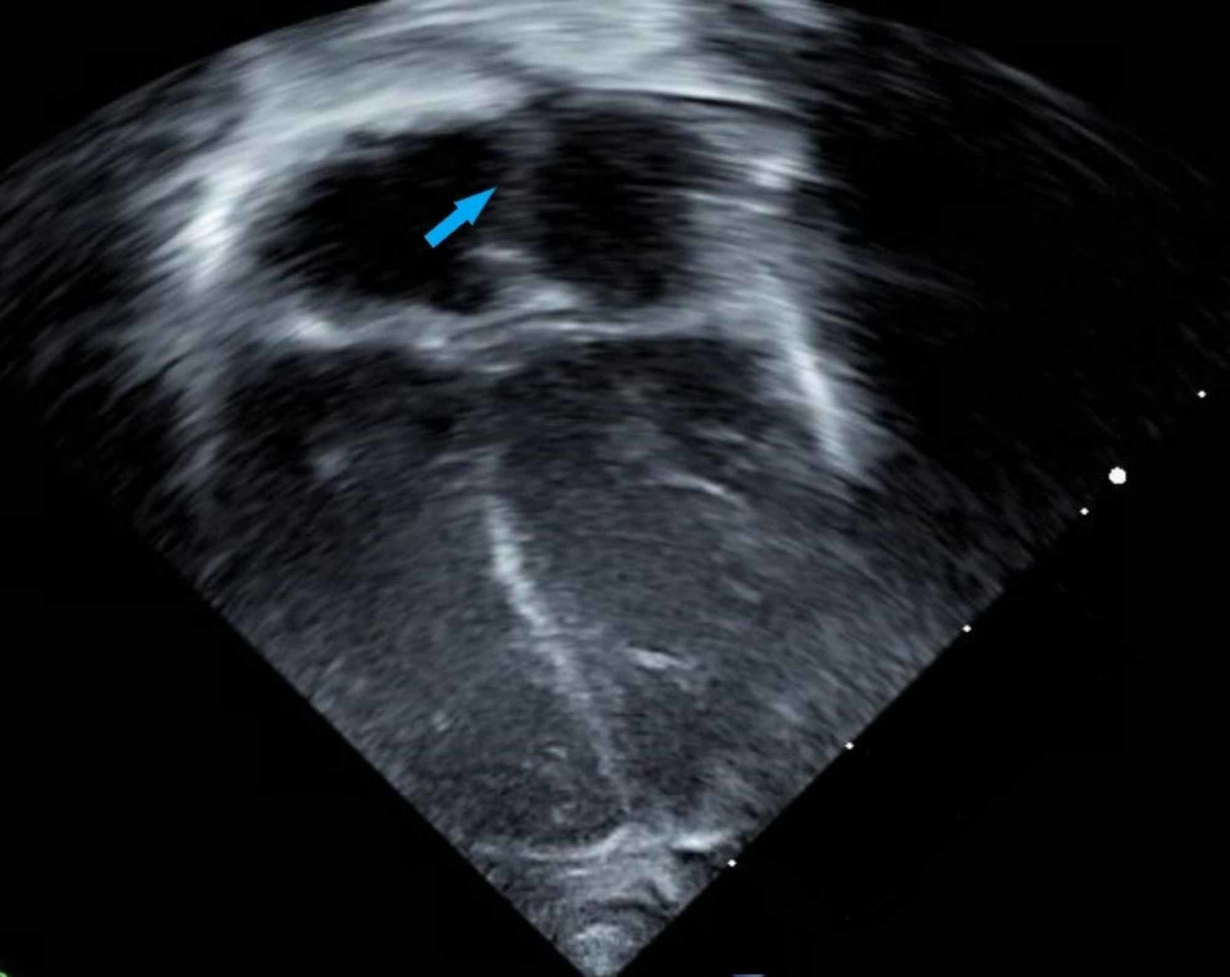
Echocardiogram apical four-chamber view showing a patent foramen ovale (arrow).
